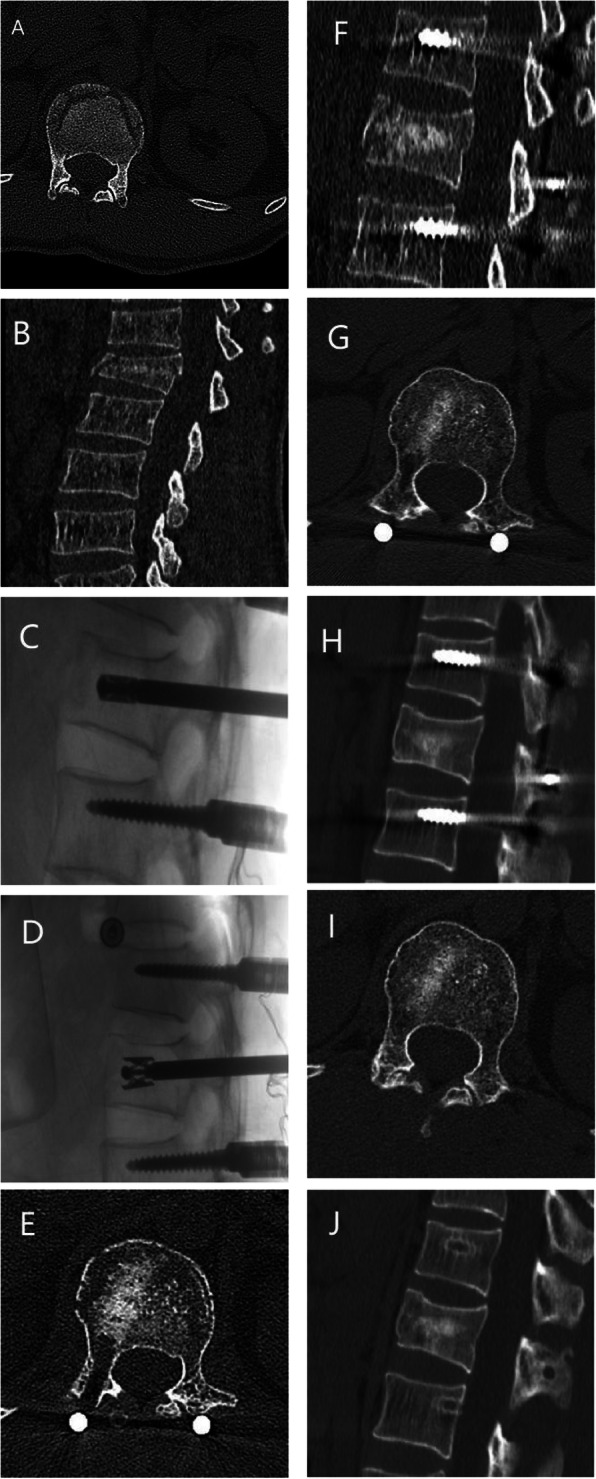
Imaging of a typical patient who underwent the novel transpedicular reducer procedure for reduction and bone grafting combined with pedicle screw fixation before, during and after the operation. A, B: Preoperative coronal and sagittal CT demonstrating a burst fracture of the vertebral body. C, D: During the operation, the new transpedicular reducer was used to reset the injured vertebra. E, F: Coronal and sagittal CT 3 days after the operation demonstrating that the allograft was evenly wedged into the anterior and medial columns. G, H: Coronal and sagittal CT 12 months after the operation. The allograft bone that filled the anterior and middle columns was partially absorbed. No defects were found, and the trabecular structure was visible in the cancellous bone. I, J: Coronal and sagittal CT after removal of the internal fixation
